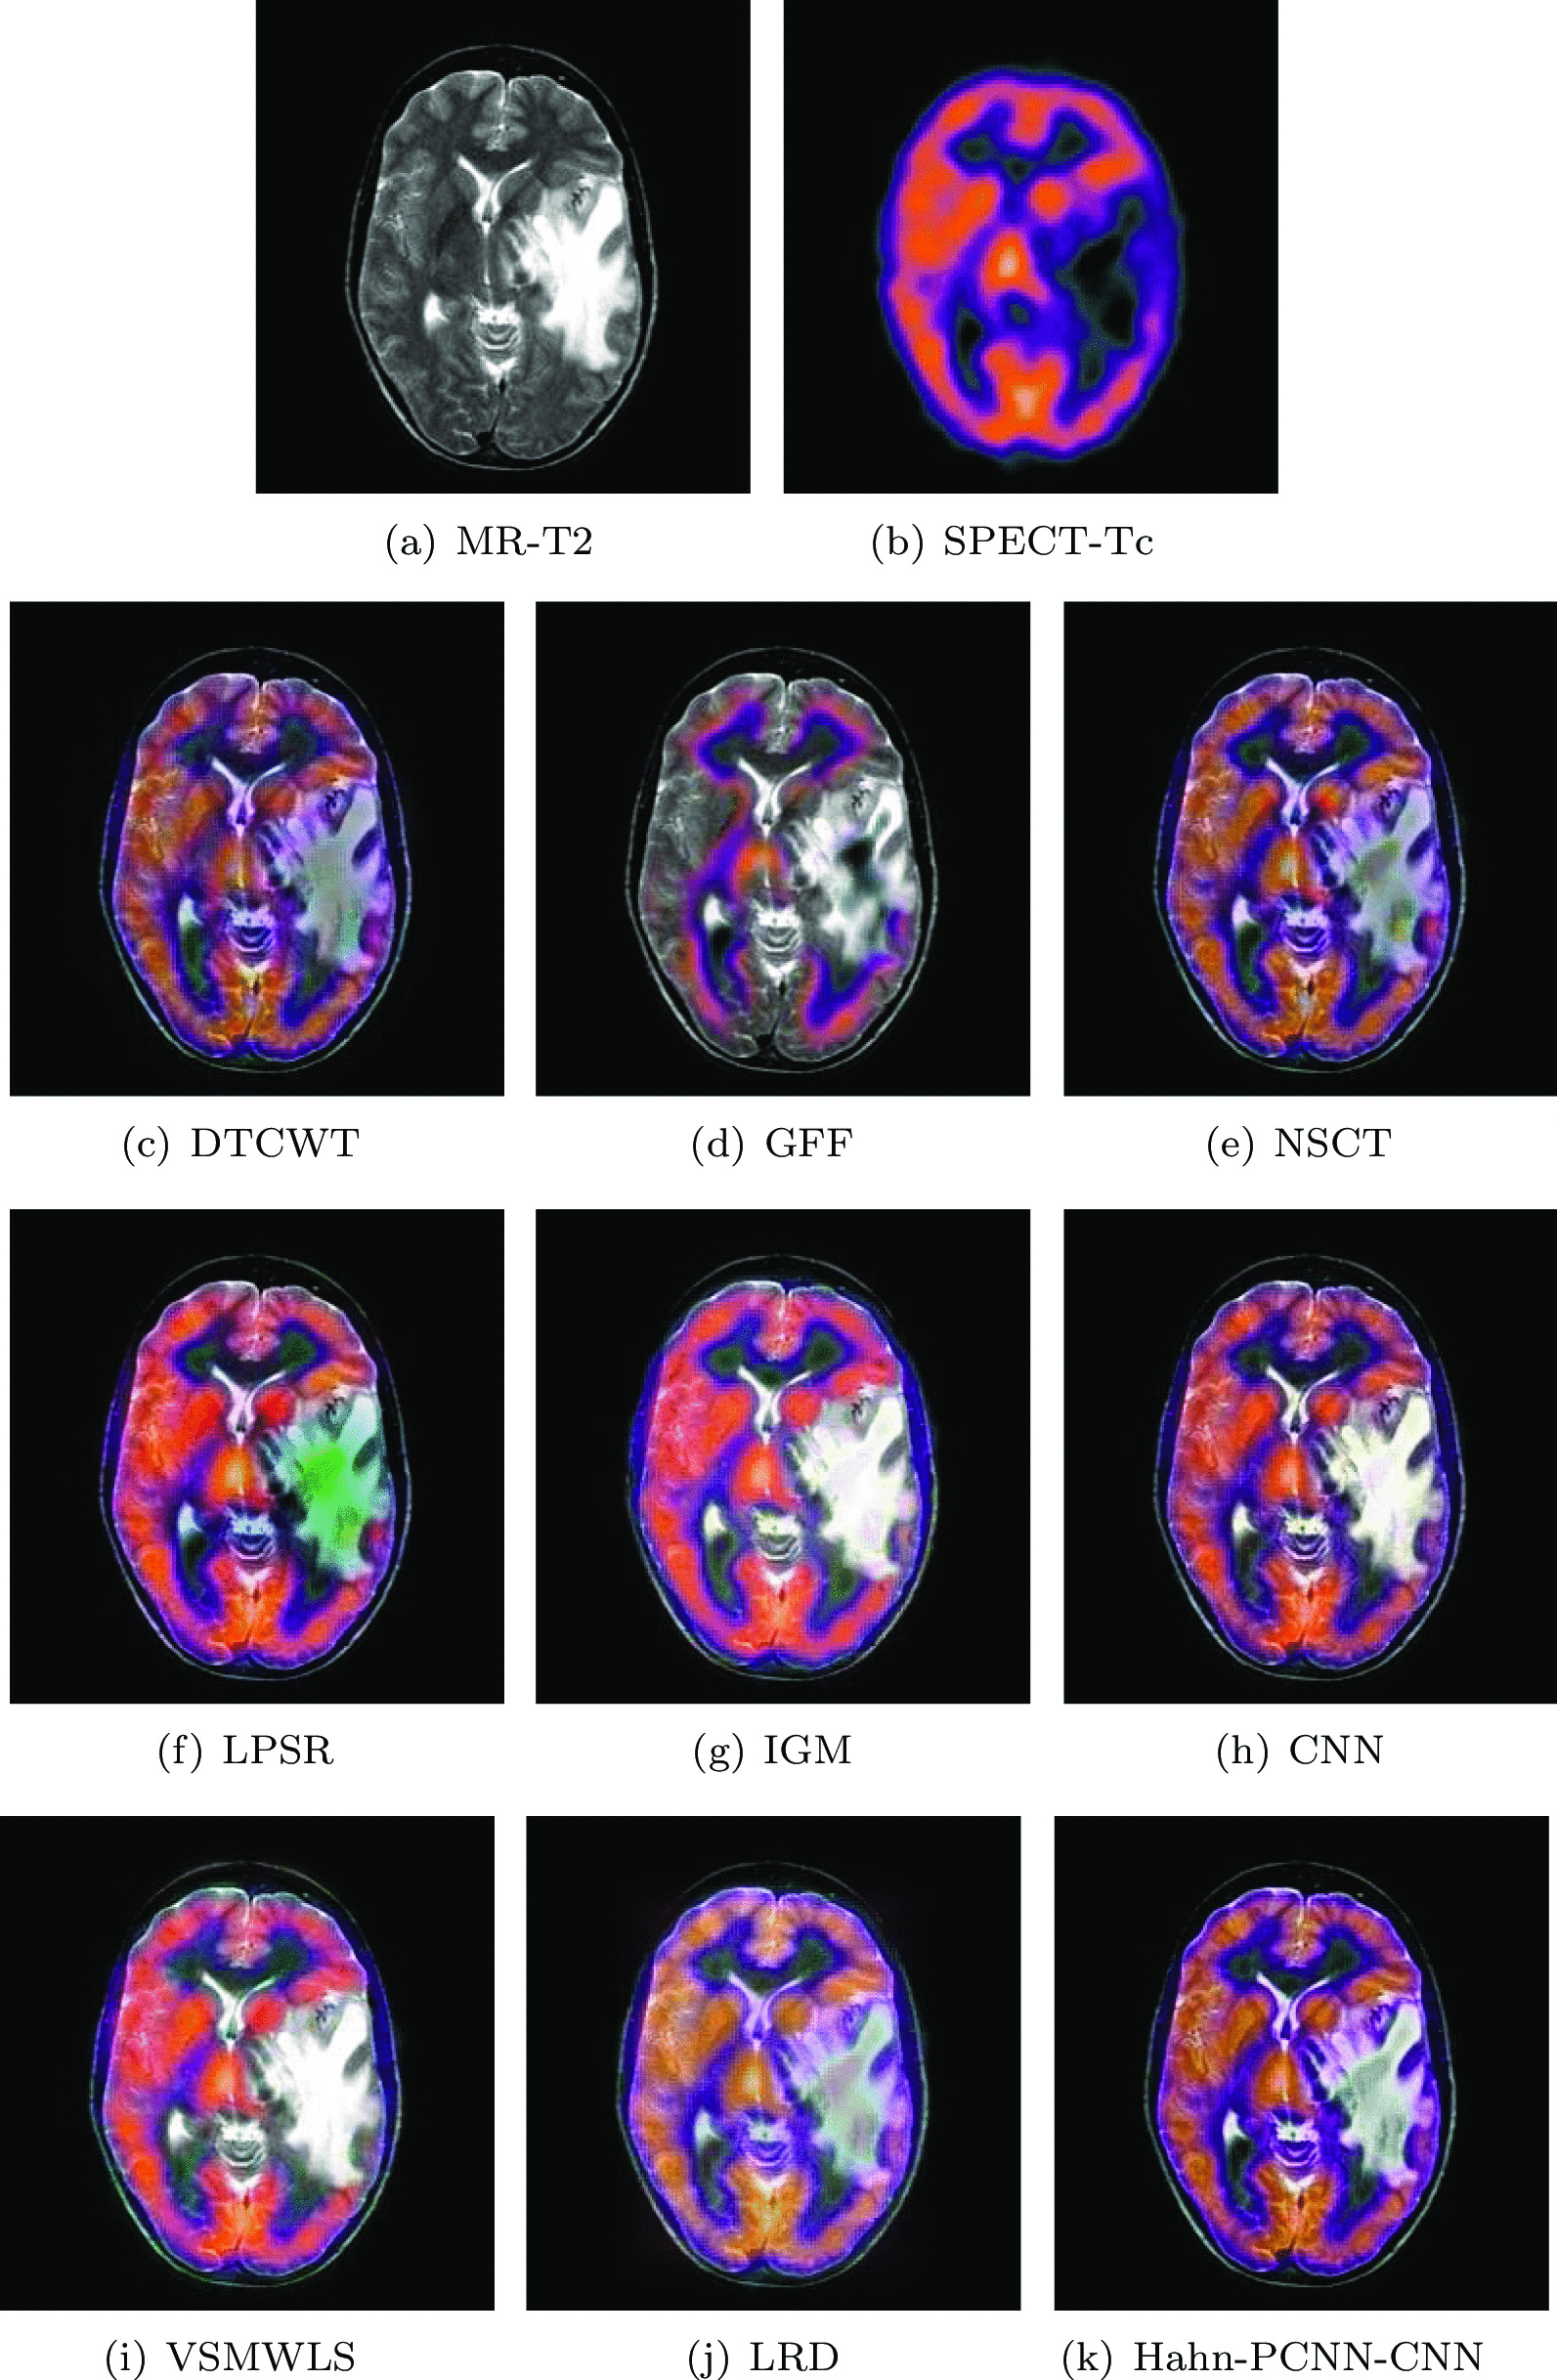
The second set of fused MRI-SPECT images from 9 methods on metastatic bronchogenic carcinoma
